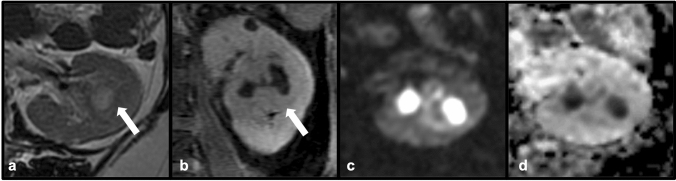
Axial T2 (a) and coronal post-contrast T1-LAVA (b) MRI demonstrates a T2 hyperintense, bilobed and thick-walled collection within the left kidney (white arrows). Increased signal on axial diffusion-weighted image (DWI) and corresponding decreased signal on absolute diffusion coefficient (ADC) map (c and d) consistent with diffusion restriction confirm the presence of a left renal abscess. This patient had known endocarditis suggesting septic embolism as the source
